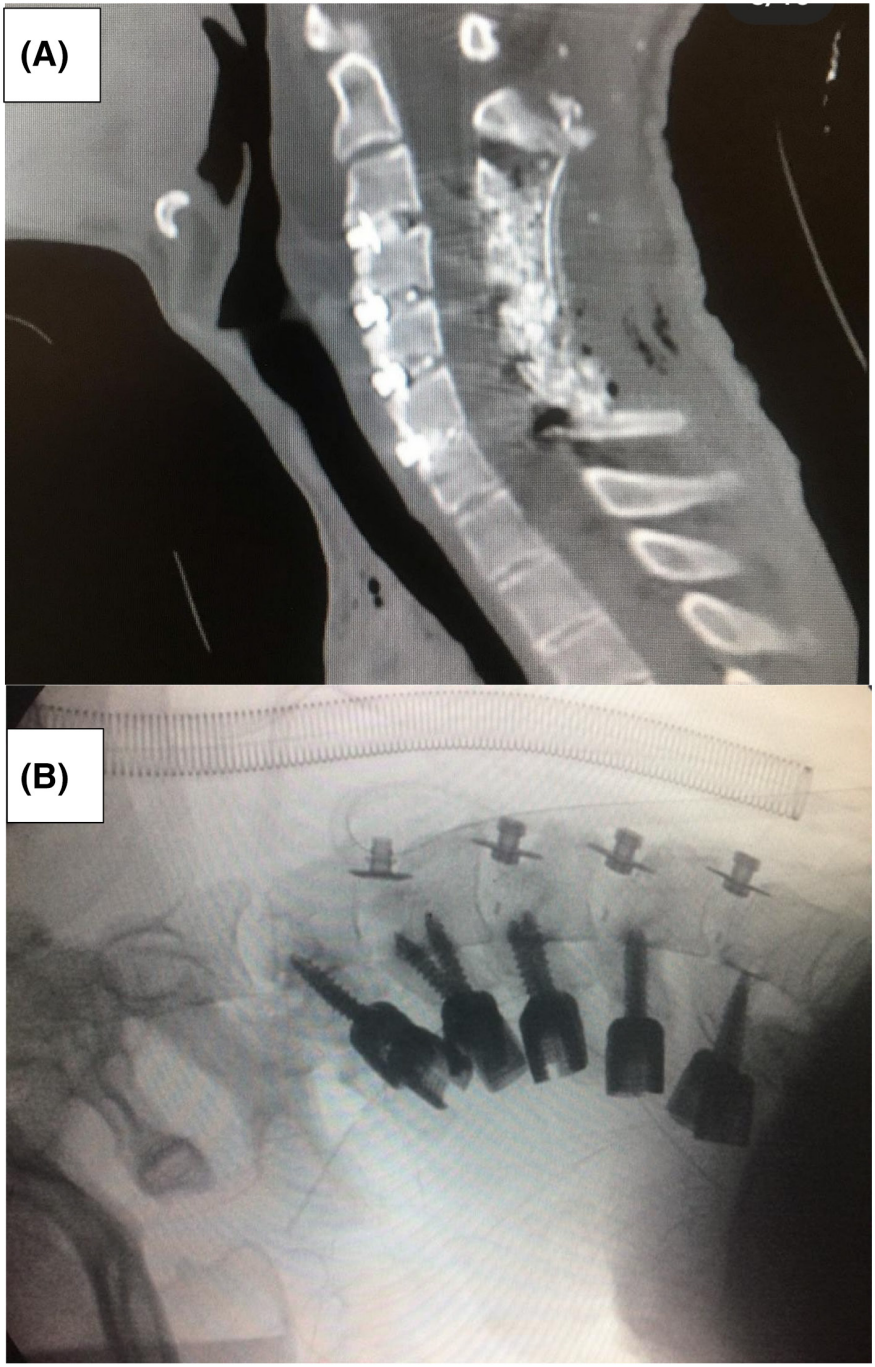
(A) Postoperative neck CT scan—sagittal view. (B) Intraoperative C‐arm fluoroscopy.
